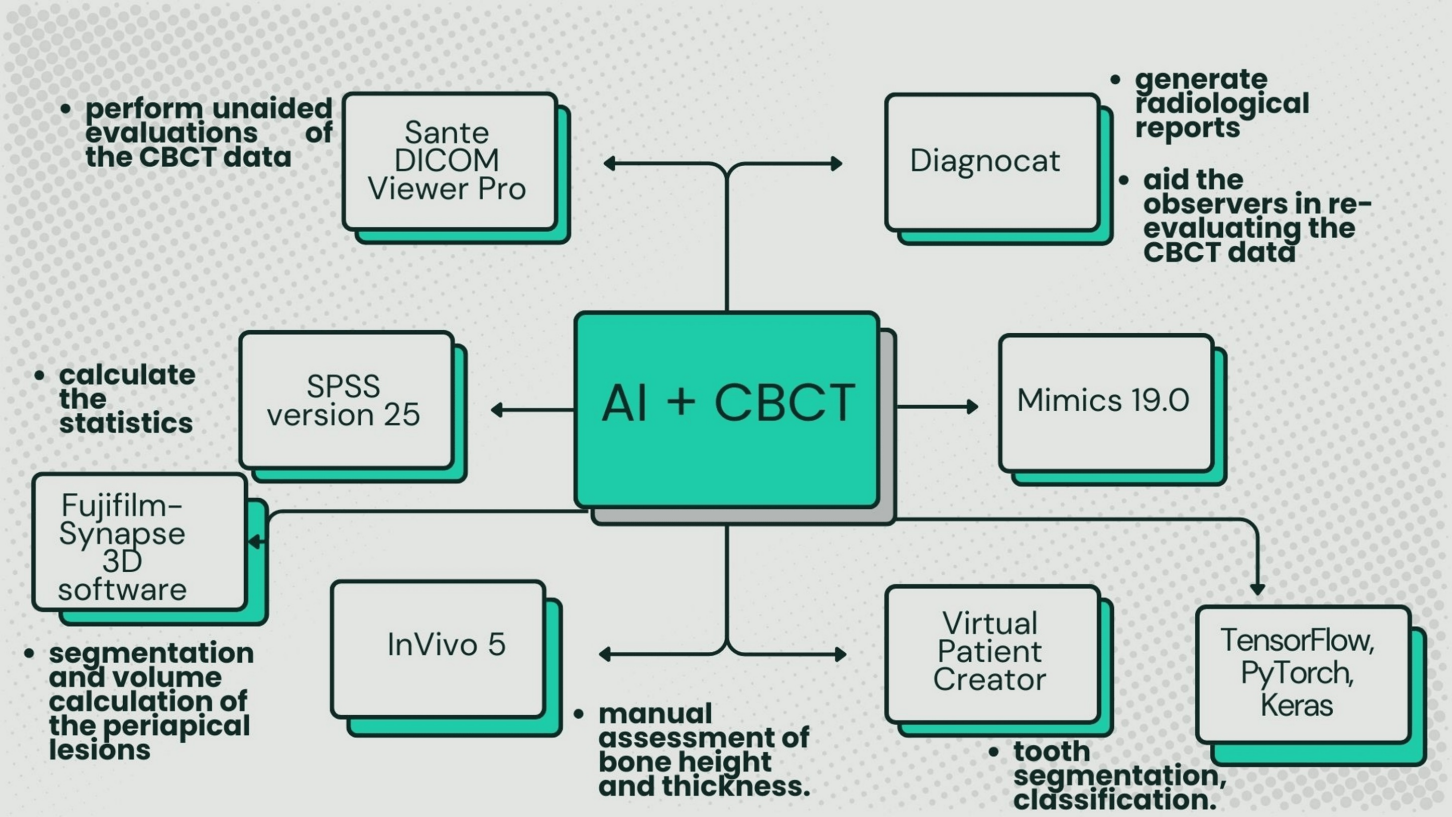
Integration of CBCT with AI. CBCT, cone-beam computed tomography; DICOM, Digital Imaging and Communications in Medicine; SPSS, Statistical Package for the Social Sciences; AI, artificial intelligence Image credits: It was created by Mrunali G. Gharat and approved by Amit Patil.
